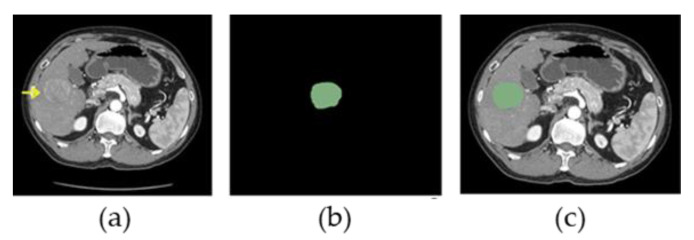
54-year-old man with hepatocellular carcinoma (HCC). (a) Original arterial phase CT scan slice shows the HCC lesion (yellow arrow). (b) ROI (green area) manually drawn in accordance with the HCC lesion. (c) Arterial phase CT scan slice shows an overlapped image of ROI (green area) and raw CT image.
